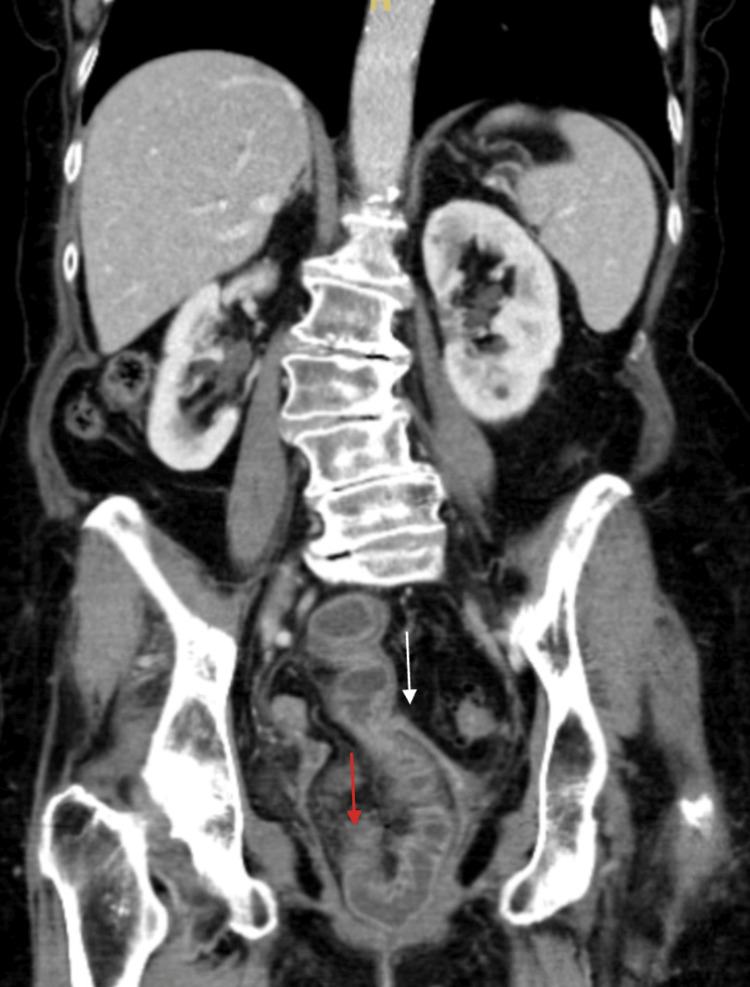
CT scan findings of vaginal cuff dehiscence. Red arrow: Dilated and thickened segment of the small bowel within the vaginal cuff. White arrow: Thickened and enlarged vaginal cuff.
